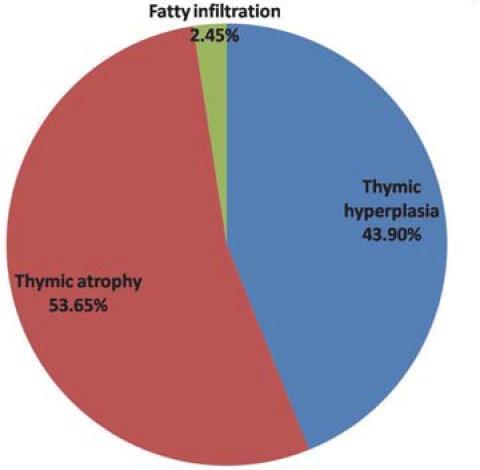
Different pathologic aspects in all patients with partial or total replacement of the thymus by fat on CT scan
